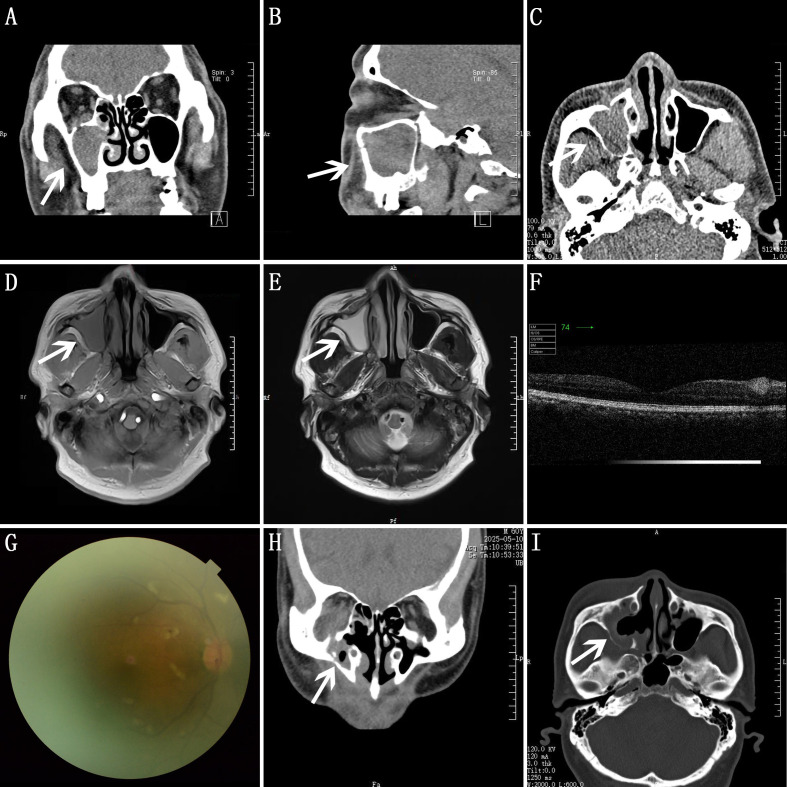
(A–C) Sinus CT findings before operation shows a slightly high-density shadow in the right maxillary sinus, with no obvious bone defect. (A) The coronal view. (B) The sagittal view. (C) The axial view. (D, E) Head MRI. (D) A short T1 signal shadow in the right maxillary sinus. (E) A long T2 signal shadow in the right maxillary sinus. (F) Optical coherence tomography of the right eye during the patient’s first hospitalization before blindness. (G) Fundus photography of the right eye during the patient’s first hospitalization before blindness. (H, I) Sinus CT findings after operation. (H) The coronal view. (I) The axial view.
